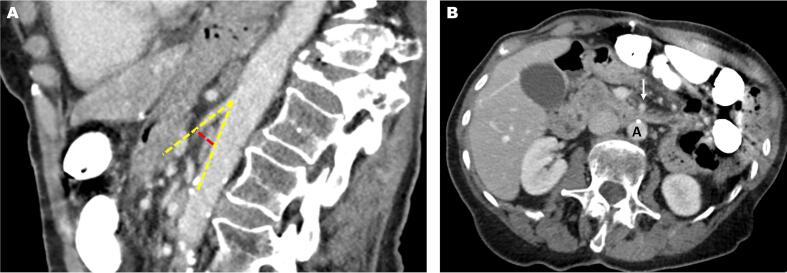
Preoperative abdominal computerized tomography (CT) images: A) Sagittal view contrast-enhanced CT shows a borderline-normal aortomesenteric with an angle of 28 degrees (dashed yellow line) and an aortomesenteric distance of 10 mm (dashed red line). B) Axial contrast-enhanced CT shows the aortomesenteric distance between superior mesenteric artery (white arrow) and aorta (A) without duodenal obstruction. (For interpretation of the references to colour in this figure legend, the reader is referred to the web version of this article.)
